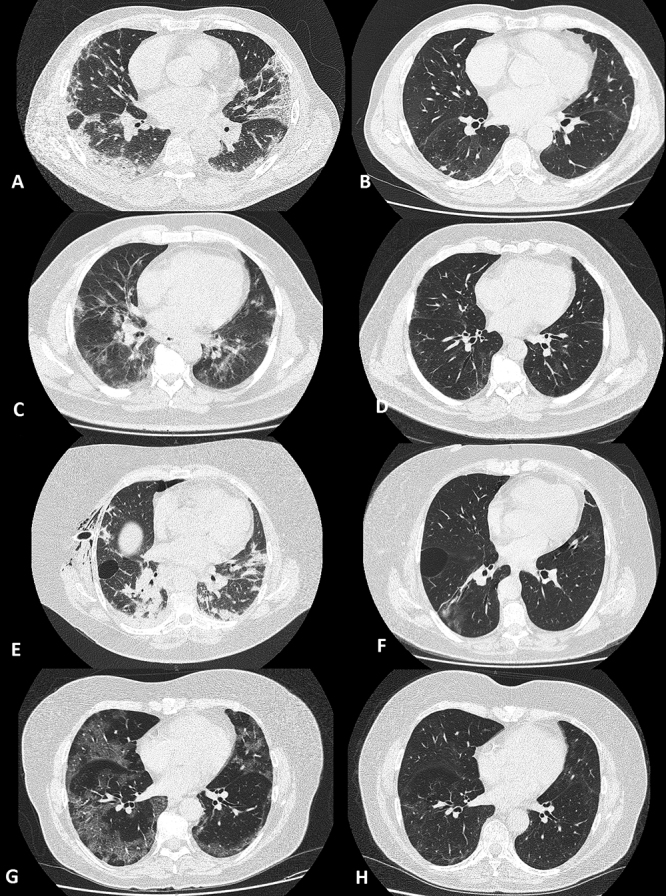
(A, C, E and G) Chest computed tomography (CT) at admission of patients 1, 2, 3 and 4, respectively. (B, D, F and H) CT 90 days after discharge of patients 1, 2, 3 and 4, respectively. All initial CT scans show bilateral, multilobar and peripheral predominance ground-glass opacities, consolidation, and septal thickening. Although all CT scans after 90 days showed a reduction in the extent and intensity of lung injury, mild ground-glass and reticular opacities with peripheral predominance remained in all patients.
